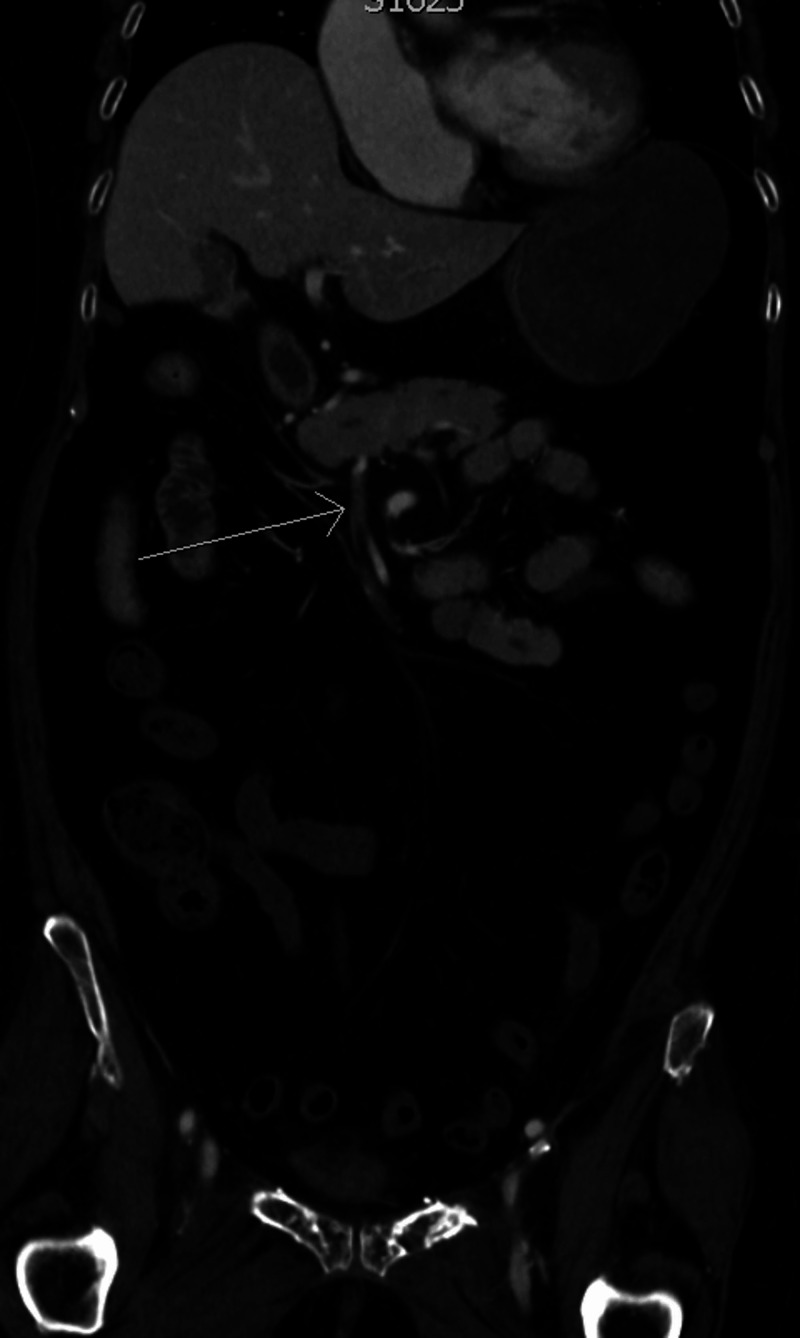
Coronal CT angiography demonstrating the superior mesenteric artery with complete occlusion with thromboembolism (arrow) and lack of distal flow
